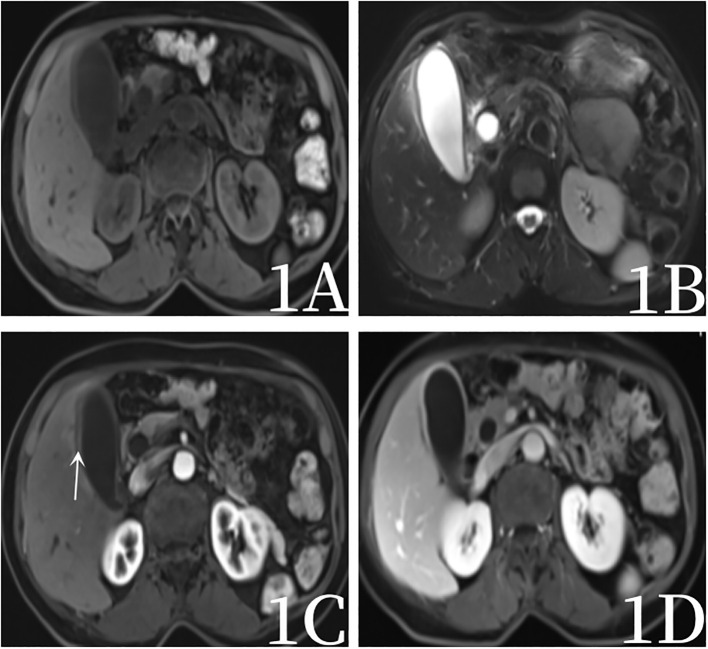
Female, 69 years old, a year ago, magnetic resonance examination, 1A (T1-weighted images), 1B T2-weighted images), 1C (arterial stage 20s), 1D (portal stage 55s) showed a slight thickening of the gallbladder wall (arrow), mild enhancement, gallbladder sediment stones, no signs of gallbladder tumor.
